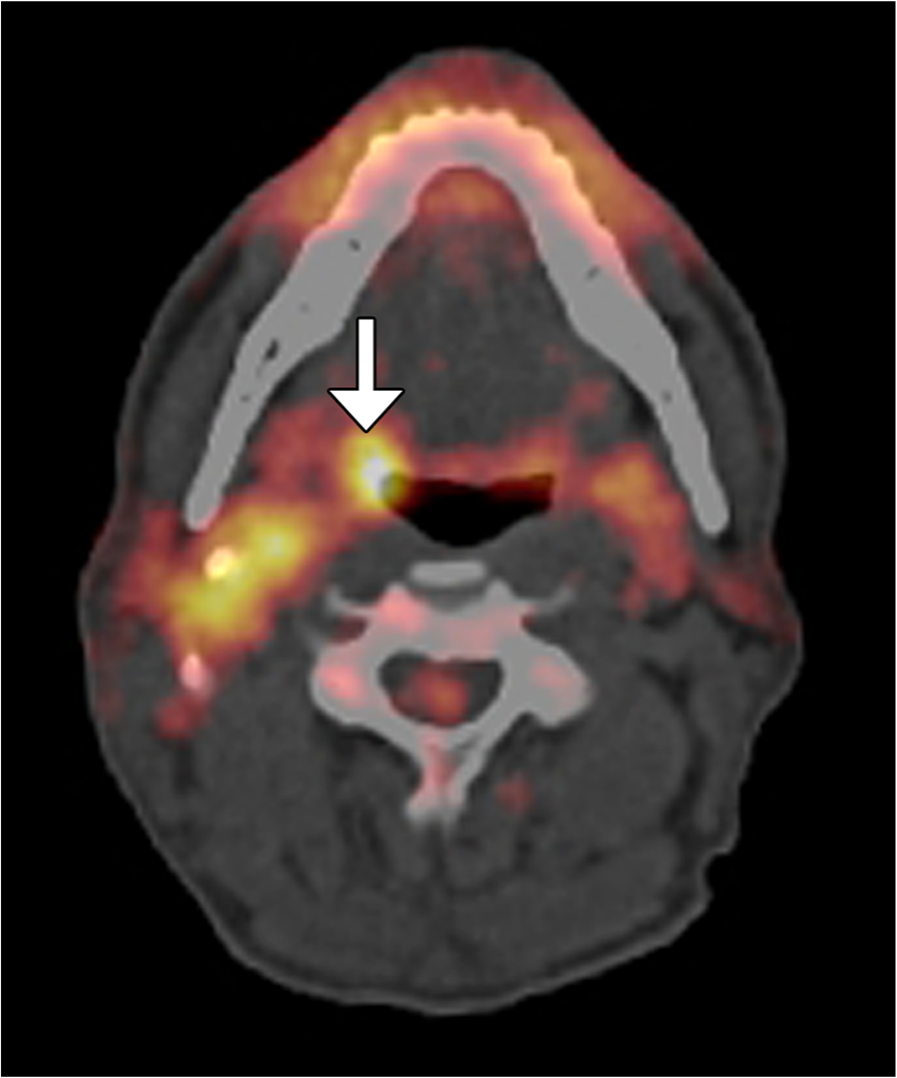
Tumor of unknown origin. ^18^FDG-PET/CT image shows right hypermetabolic cervical lymphadenopathy and a small ipsilateral hypermetabolic oropharyngeal lesion that proved to be the primary squamous cell carcinoma, which was not initially found on endoscopy
